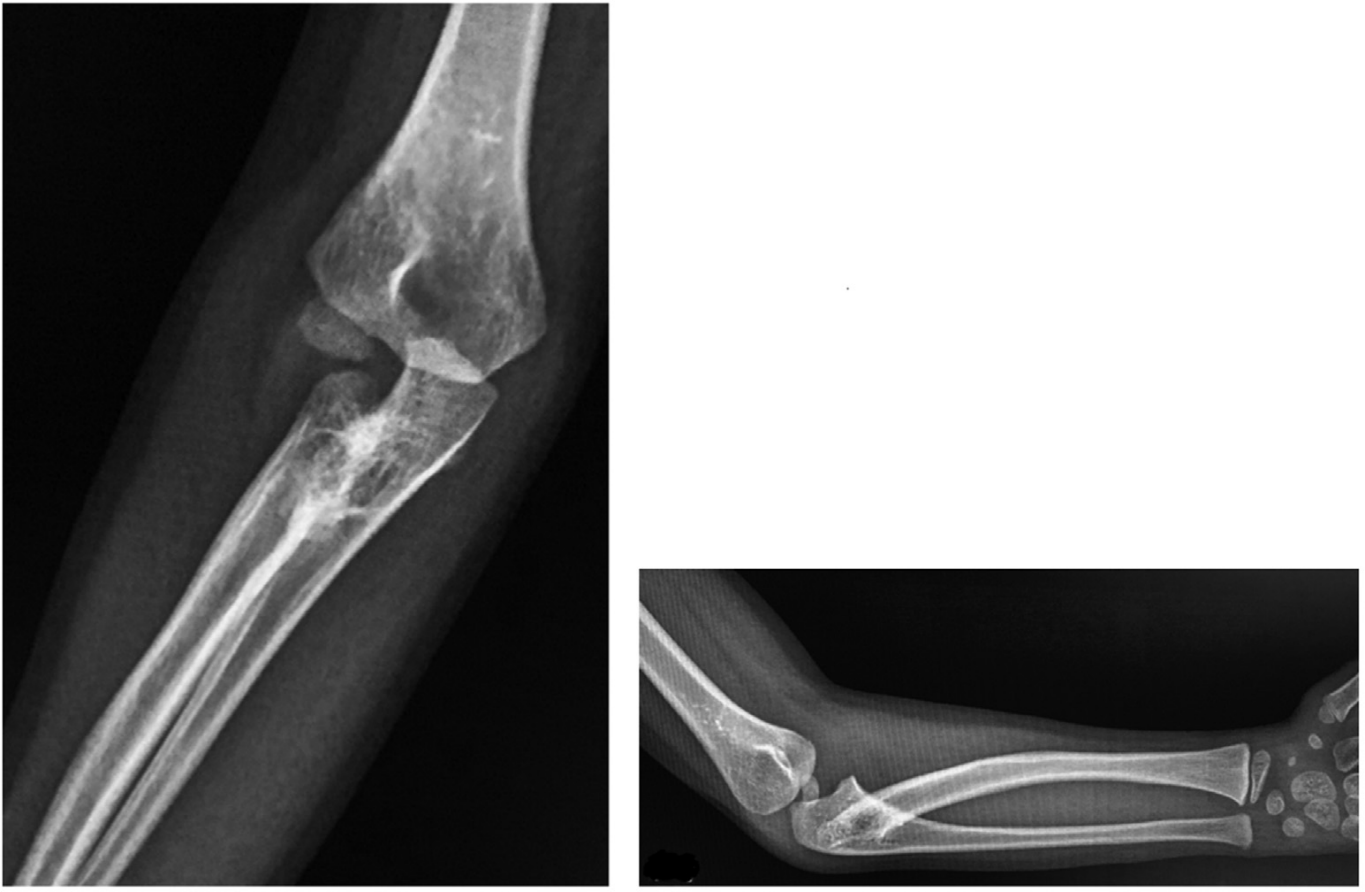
Radiographs of the right elbow showed proximal radioulnar synostosis, posterior radial head dislocation, and posterior bowing of the radius.
